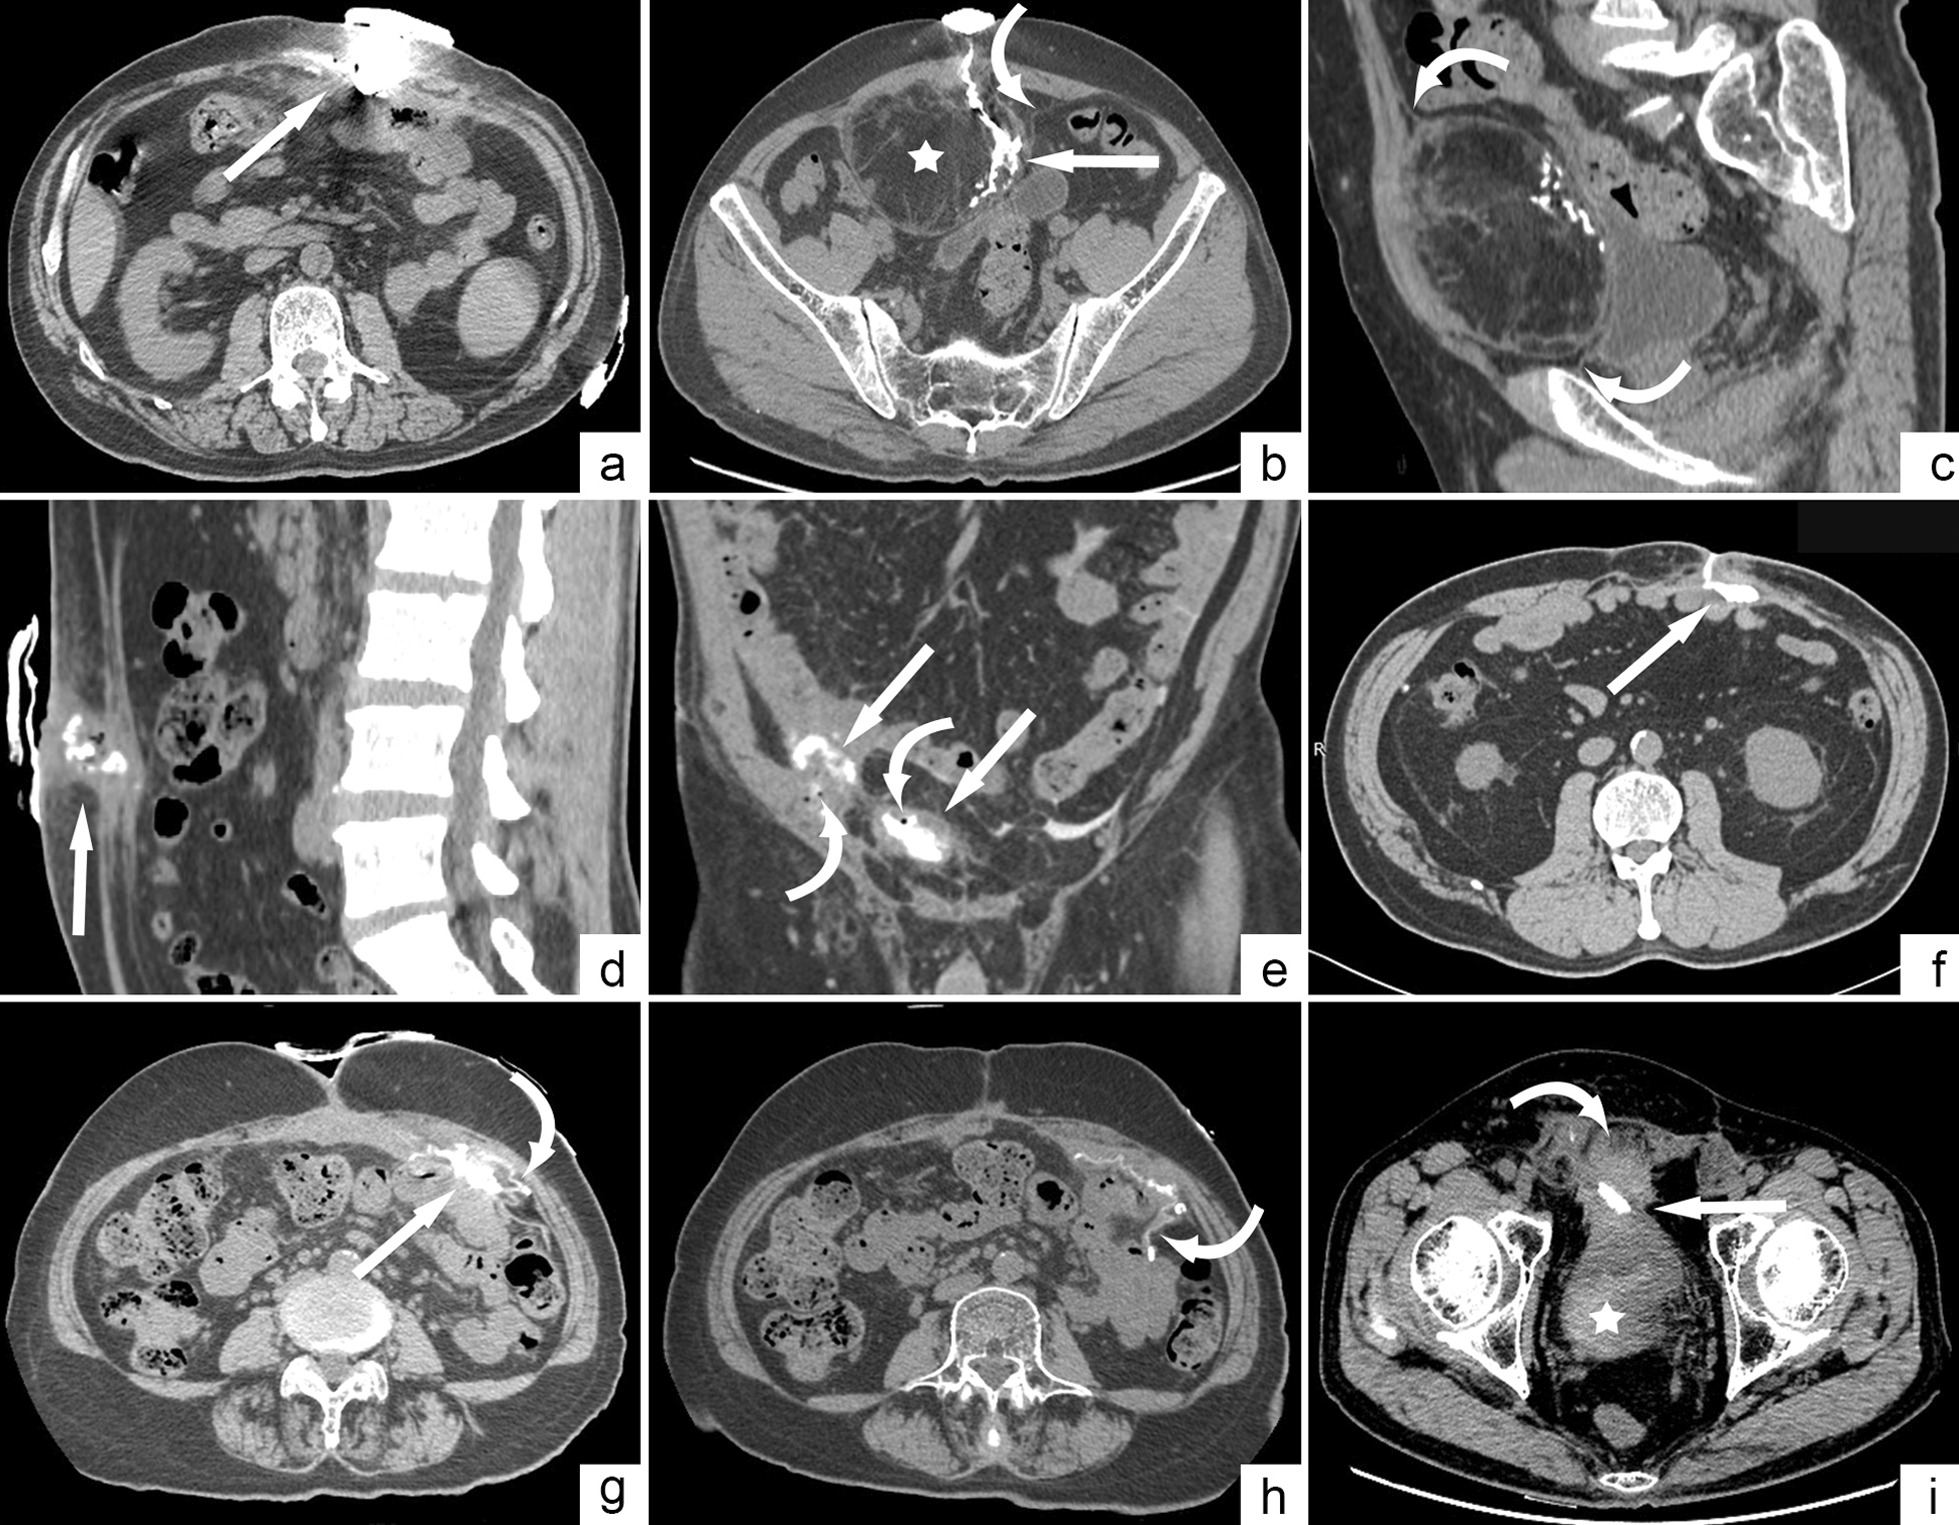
Missed diagnosis and misdiagnosis cases of CT sonography. a Case 1 (sinus confined to the abdominal wall), a 56-year-old male, CT shows that the abscess cavity protrudes into the abdominal cavity (arrow). b, c Case 2 (sinus tract confined to the abdominal wall), a 65-year-old male, the sinus runs in a strip antero-posteriorly (arrow), and a mass formed by fat necrosis (☆) can be seen in the adjacent abdominal wall. The adjacent peritoneum appears intact (curved arrow). d Case 5 (sinus involving the abdominal cavity), a 66-year-old man, the sinus is mainly located in the abdominal wall on CT image (arrow). The adjacent peritoneum is thickened, but its integrity could not be judged. e Case 6 (sinus involving the abdominal cavity), a 71-year-old man, the sinus involves the abdominal cavity, with deep abscess (arrow) containing many small bubbles (curved arrow), leading to misdiagnosis of ECF. f Case 7 (ECF), an 82-year-old female. Most of the lesion is in the abdominal wall (arrow) and closely adhered to the adjacent intestinal wall. There are no signs of ECF. g, h Case 8 (ECF), a 64-year-old male, the sinus involves the abdominal cavity, and the adjacent mesh is thickened and curled (curved arrow). No signs of ECF are found. i Case 4 (sinus confined to the abdominal wall), a 32-year-old female, the abdominal wall around the sinus is obviously thickened (curved arrow), and the border with the anterior bladder wall is indistinct (arrow). The bladder density increases up to 76HU due to entry of the contrast agent (☆), which lead to a misdiagnosis of vesico-cutaneous fistula. *CT* computed tomography, *ECF* enterocutaneous fistula
